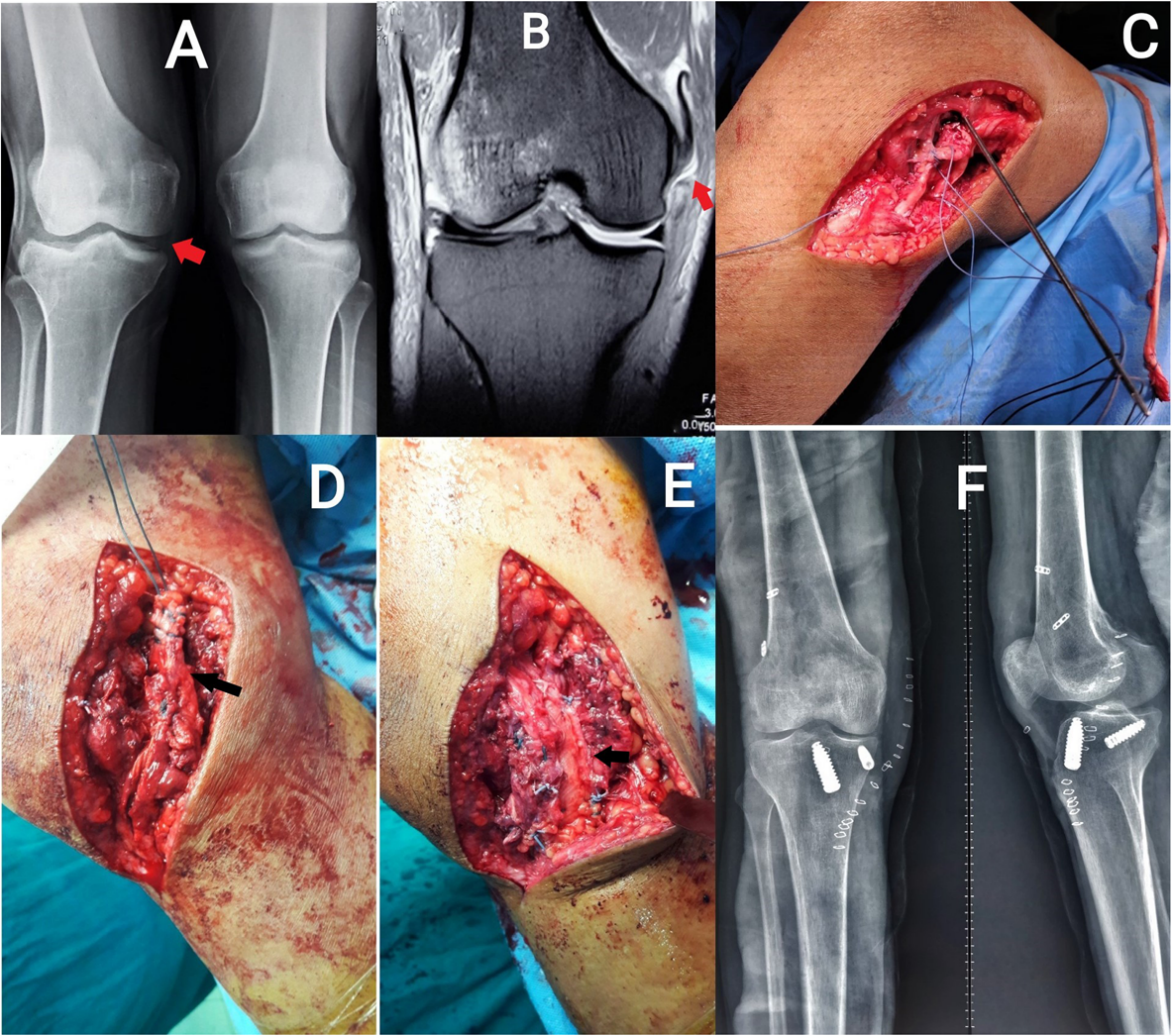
a Preoperative stress radiograph of bilateral knee showing opening of medial joint space of right knee more than left side, suggesting right medial collateral injury. b Preoperative MRI picture showing MCL injury. There is discontinuity of MCL with the distal part of the ligament retracted cephalad. c Intraoperative image showing Beath pin placement for drilling of femoral tunnel for MCL reconstruction. d, e Intraoperative pictures showing graft placement. The semitendinosus graft was left attached to the tibia distally and secured in the femoral tunnel. The free end of the graft was used for reconstruction of the POL. f Postoperative X-ray of the same patient showing fixation of the ACL, MCL, and POL with interference screws and adjustable-length suspensory button
